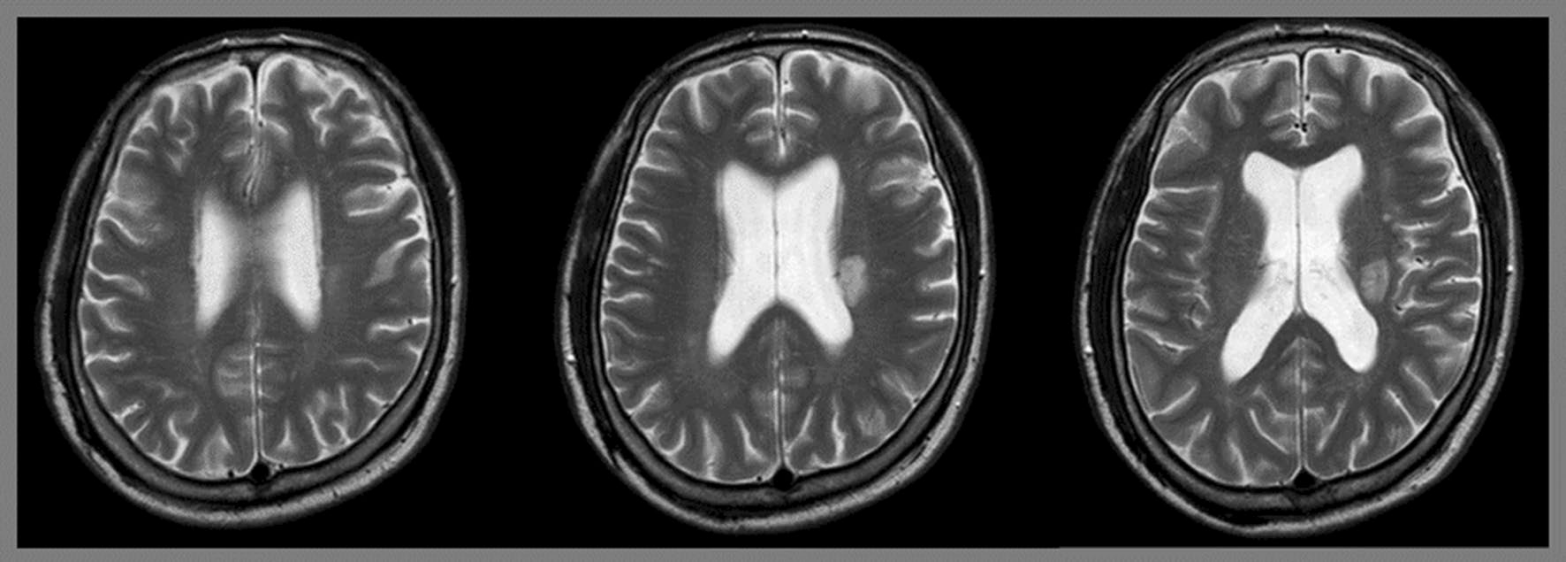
Three axial consecutive brain T2-magnetic resonance images from a 67-year-old man with left corona radiata infarct.
